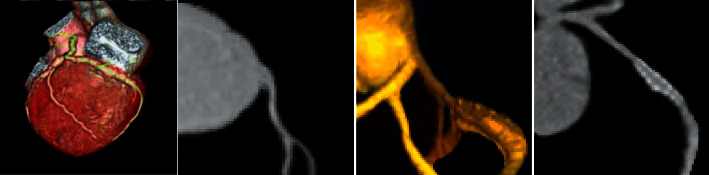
CTA images of a patient. The images from left to right were CTA cardiac image, coronary CTA image, coronary CTA image, and cervical vascular image, respectively.
